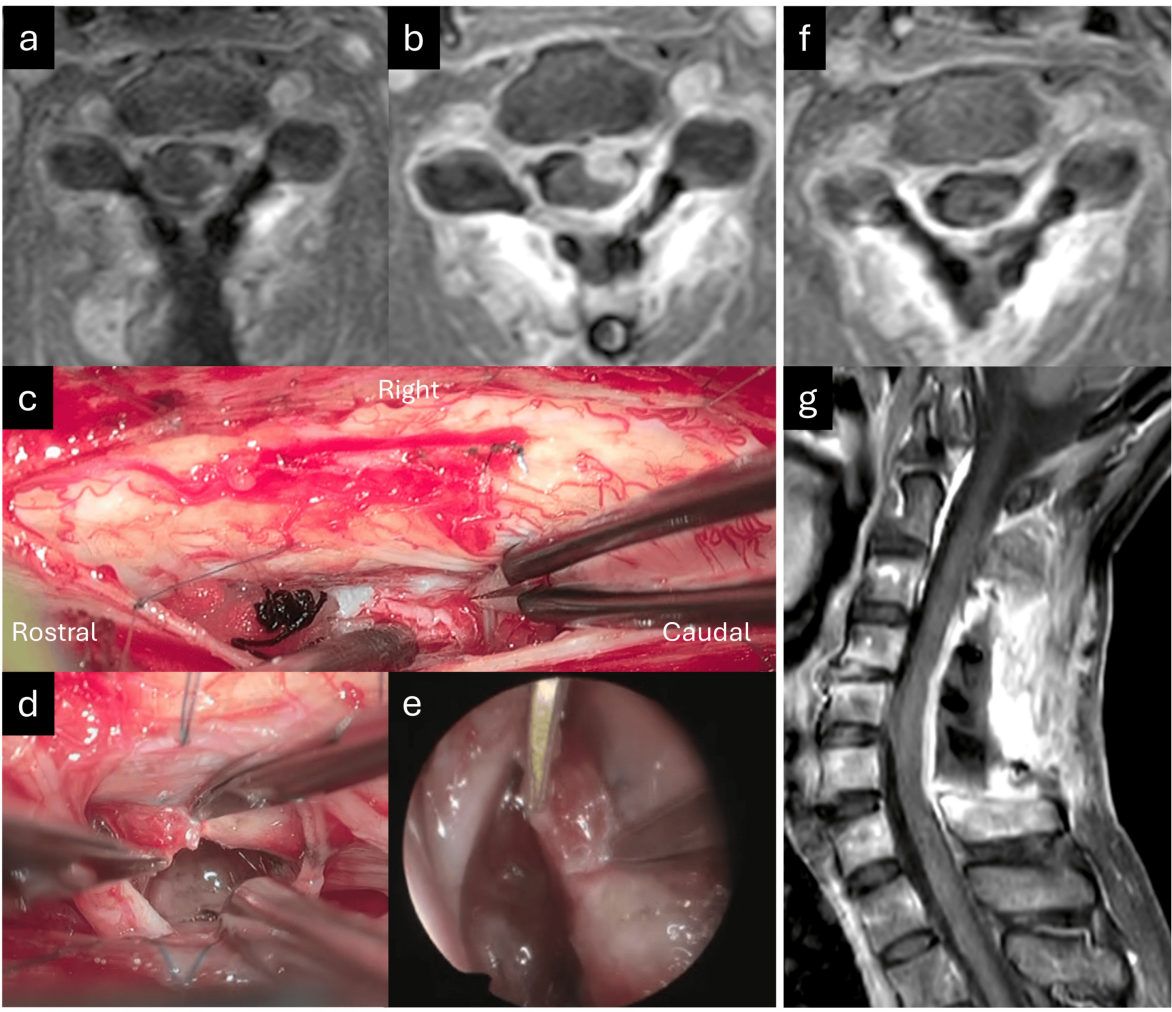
Preoperative, intraoperative, and postoperative findings at the second surgery (a) Axial contrast-enhanced gadolinium (Gd) magnetic resonance imaging (MRI) after the first surgery showing a small residual tumor ventrolateral to the cervical spinal cord. (b) Axial Gd MRI two months after the first surgery showing an enlarged residual tumor. (c) Intraoperative exoscopic view showing the recurrent tumor ventrolateral to the spinal cord. (d, e) Intraoperative exoscopic view and endoscopic view showing resection of the residual tumor adhesive to the ventral spinal cord with the simultaneous multi-angle view. (f, g) Postoperative axial and sagittal Gd MRI showing total removal.
